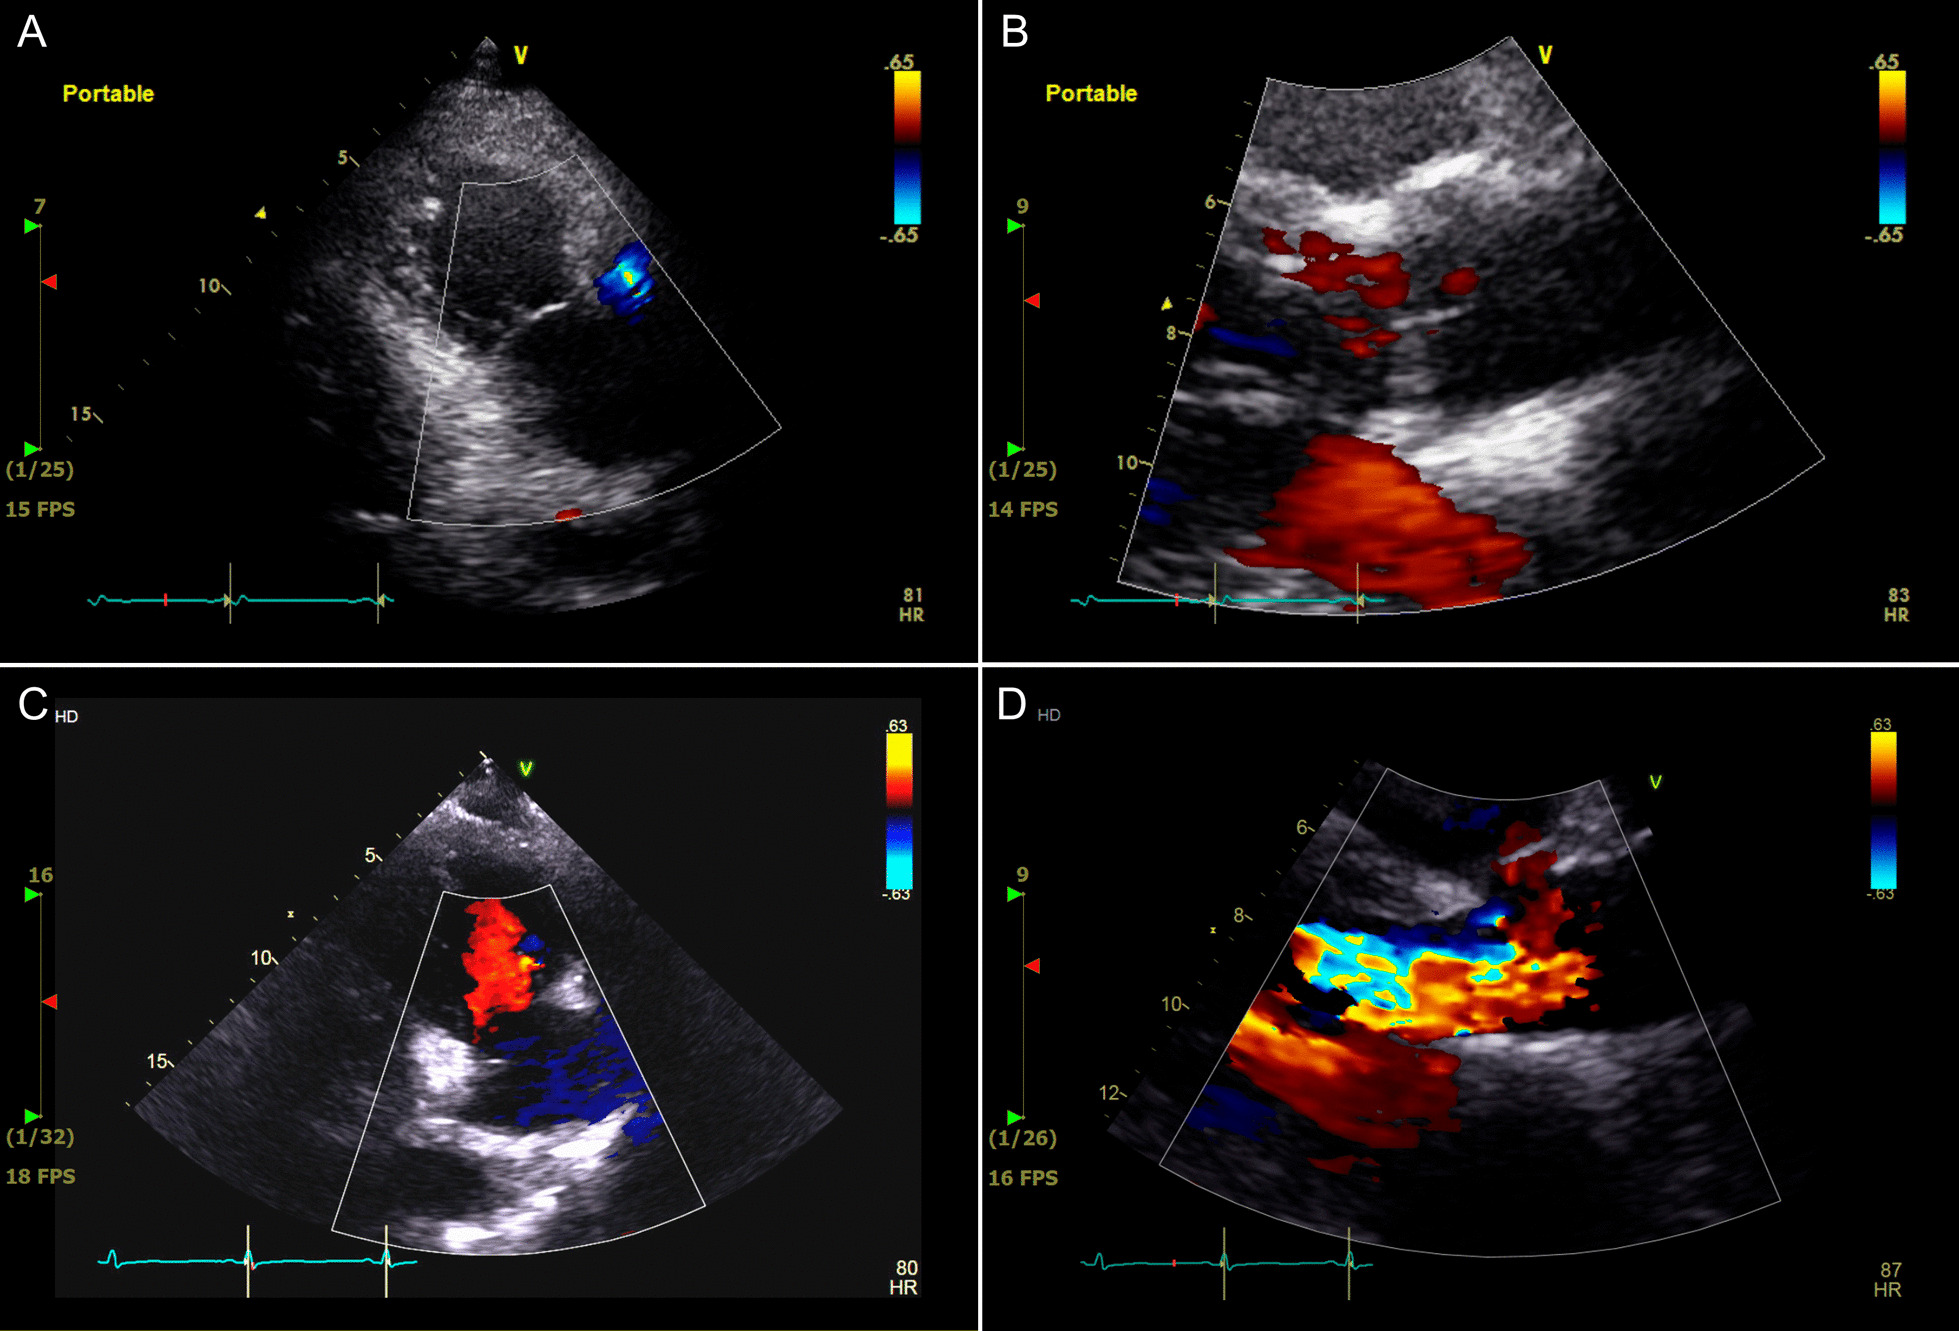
Echocardiograms performed at the time of aorta injury and 42 months after stent graft insertion. A–B An echocardiogram performed on the fourth day after TEVAR (EF 61%, LVEDD/LVESD 51/34mm, LA 40 mm, LV mass index 107.5 g/m2, Aortic annulus/Sinus valsalva/Sinotubular junction/Ascending aorta 22 mm/38mm/31mm/38mm, Trivial MR, Trivial TR). C–D An echocardiogram performed at the emergency room for sudden dyspnea 42 months after stent graft insertion (EF 63%, LVEDD/LVESD 90/46mm, LA 59 mm, LV mass index 169.7 g/m2, Aortic annulus/Sinus valsalva/Sinotubular junction/Ascending aorta 22 mm/35mm/27mm/ 40 mm, AR grade 4, MR grade 1, Trivial TR). (*EF* Ejection fraction, *LVEDD* Left ventricular end diastolic dimension, *LVESD* Left ventricular end systolic dimension, *LA* Left atrium, *LV* Left ventricle, *MR* Mitral valve regurgitation, *TR*  Tricuspid valve regurgitation,  *AR* Aortic valve regurgitation)
